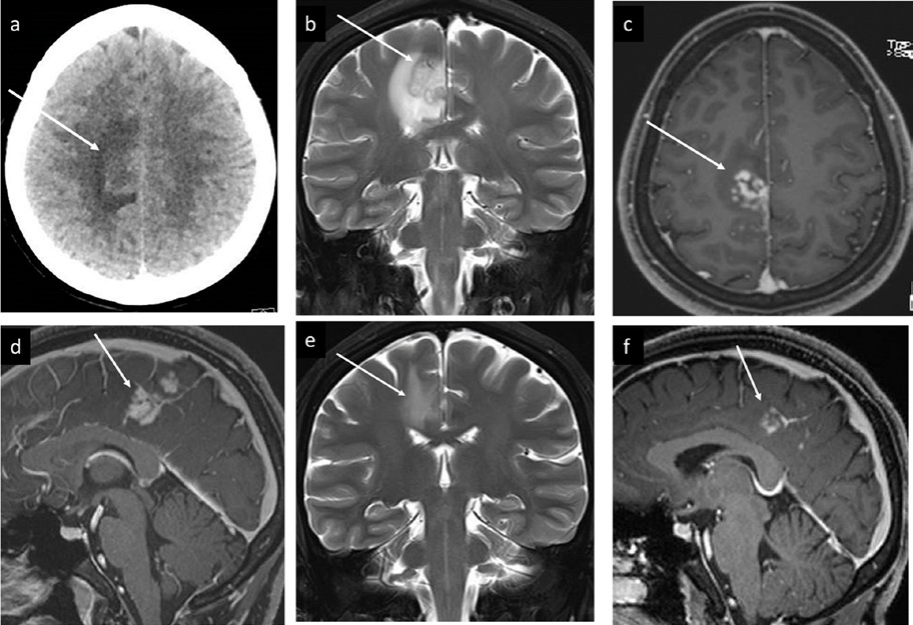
Case 2 Brain schistosomiasis, (a) Plain CT head showing left frontal parasagittal cortical swelling, and subcortical vasogenic oedema (arrow), (b) MRI Coronal *T*_2_W image,showing corresponding Gyral swelling low signal, and surrounding subcorticalvasogenic edema, (c, d) sagittal, and axial post-contrast T1W image respectively,showing the typical arborized palm tree-like enhancement. 2 weeks Post-treatment follow-up MRI showed dramatic improvement with decreased edema on coronal *T*_2_W image (e), and decreased enhancement on sagittal post-contrast *T*_1_W image (f).
